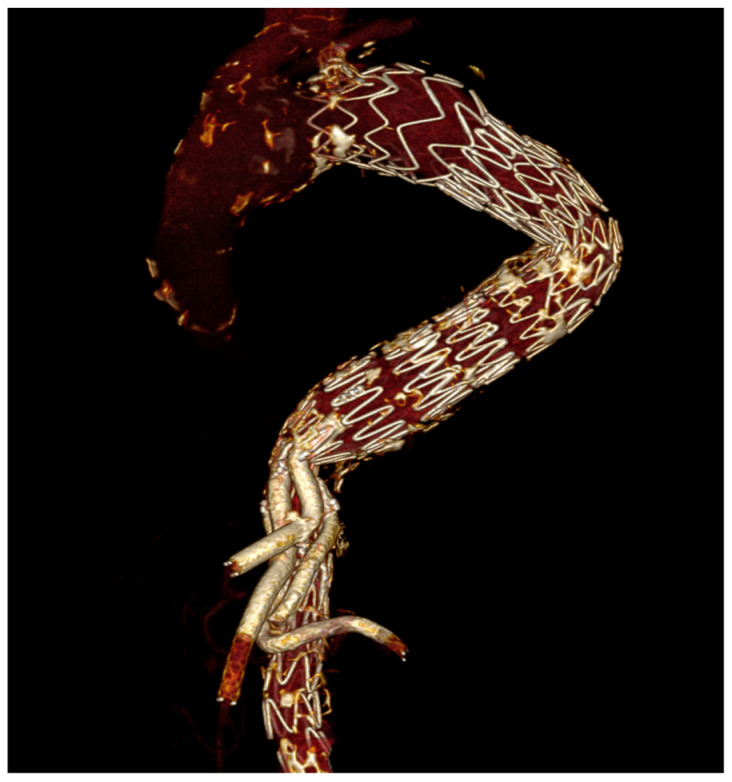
Postoperative 3D CT reconstruction following Cratos implantation combined with branched endovascular aortic repair (BEVAR).
